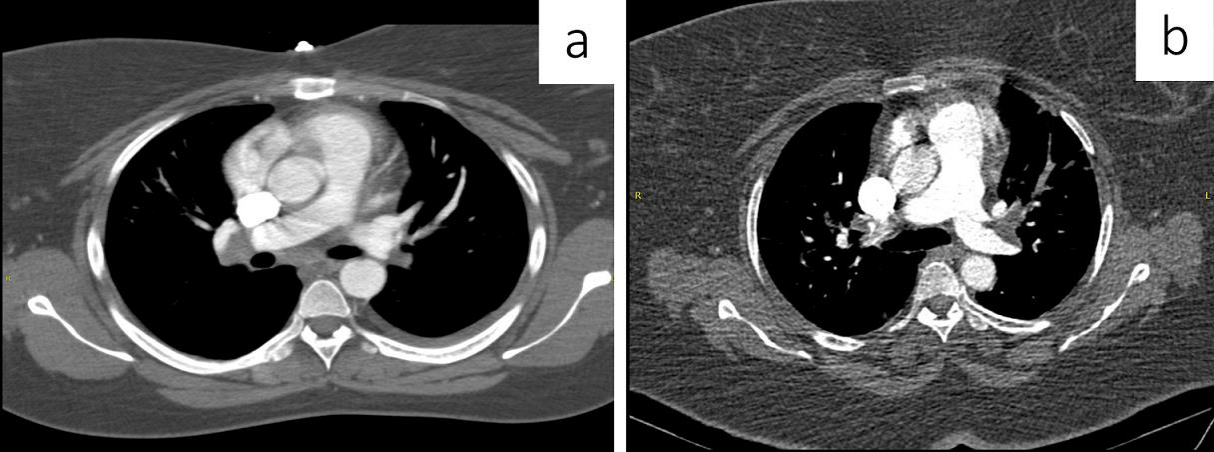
a) axial CT soft tissue window demonstrating bilateral central pulmonary emboli; b) axial CT soft tissue window demonstrating bilateral central pulmonary emboli
